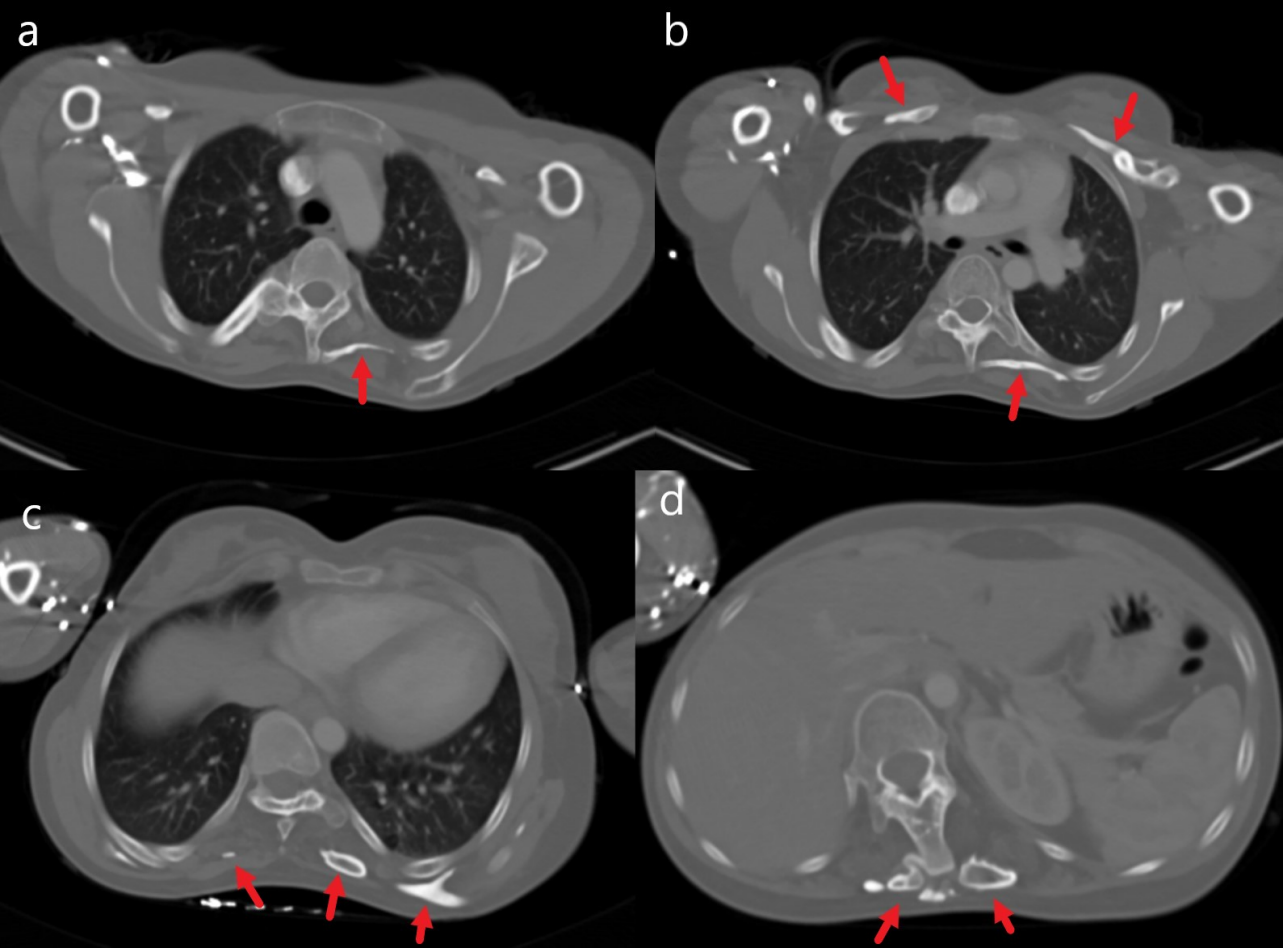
Axial CT images show heterotopic ossifications in the paravertebral and the perithoracic areas; a) upper thoracic level; b) subcarinal level; c) lower thoracic level; d) upper abdominal level
